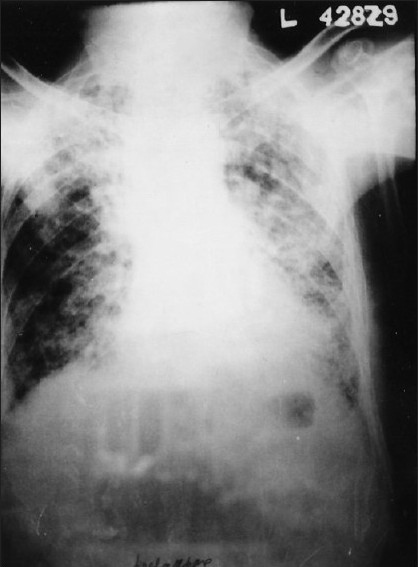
X-ray chest, PA view, showing bilateral inhomogeneous infiltrates, more on the left side
